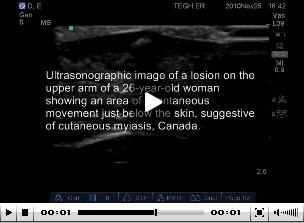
Ultrasonographic image of a lesion on the upper arm of a 26-year-old woman
